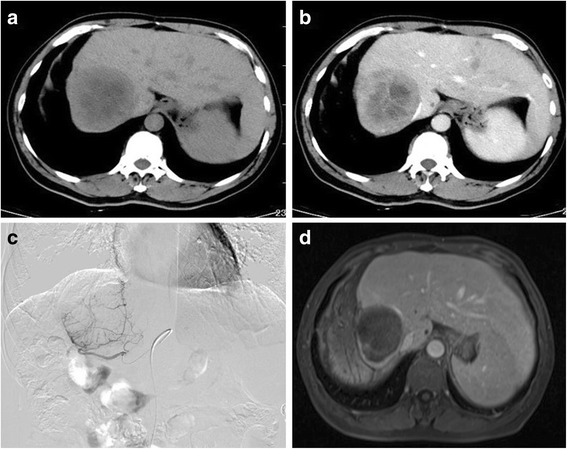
Phases of DEB-TACE with CBDOX in a 54-year-old male patient. a–b Plain phase and portal phase of pretreatment CT scan revealed a recurrence lesion located at the right lobe of the liver 11 months after surgical resection. c Angiography during the DEB-TACE procedure revealed a comparatively hypervascular lesion. d An enhanced MRI scan performed at 3 months after treatment showed a complete remission to treatment
